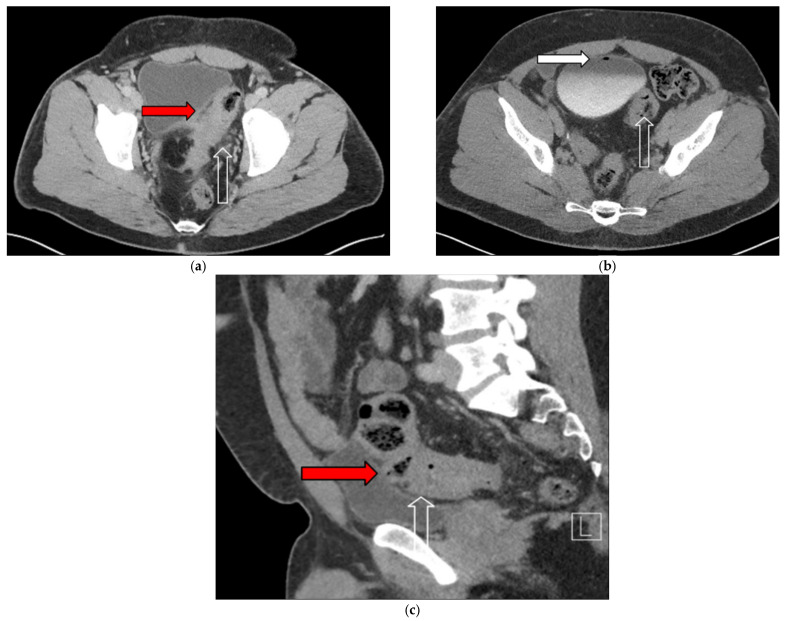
(a–c) Contrast-enhanced computed tomography images demonstrating inflammatory changes involving the sigmoid colon and bladder wall (red arrows), suggestive of colovesical fistula: (a,c) show inflammatory stranding between the sigmoid colon and bladder wall and (b) shows intravesical air (white arrow). Sigmoid colon with thickening consistent with diverticulitis is noted in all panels (white outlined arrows).
